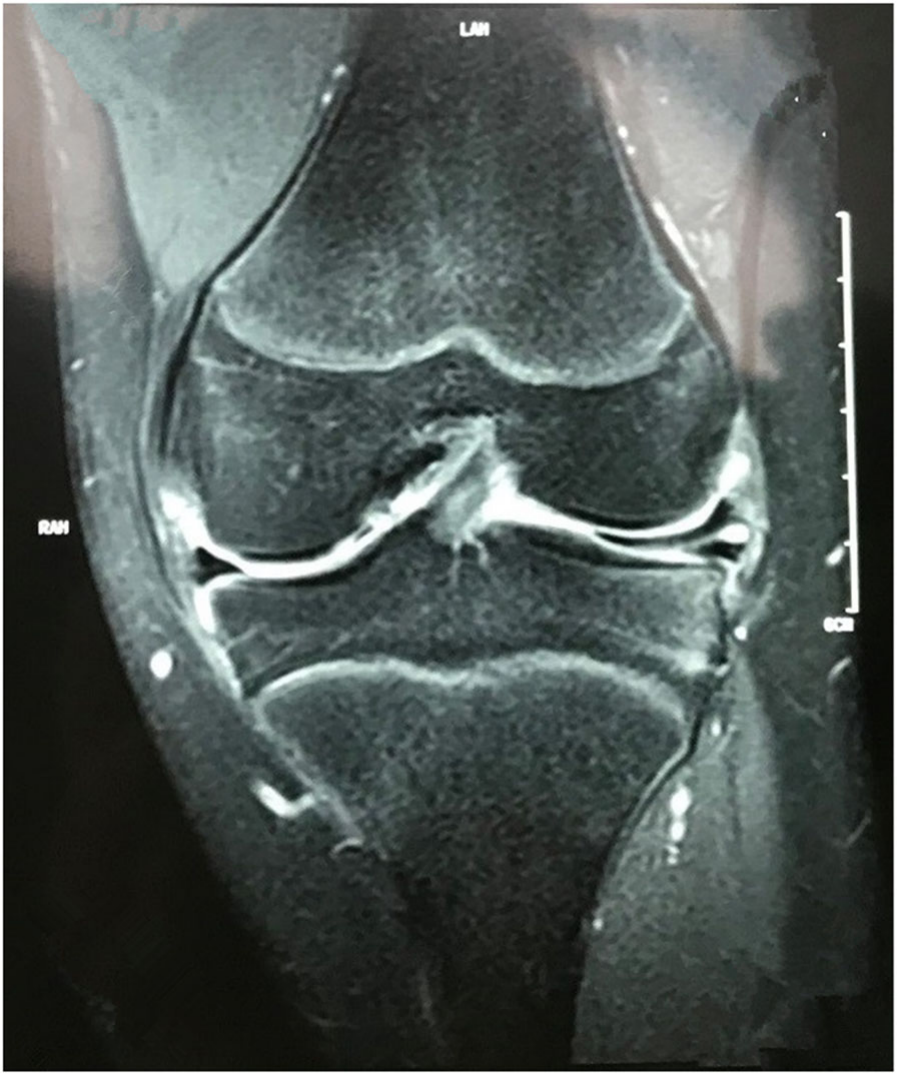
A horizontal tear of the lateral menisci, which is identified with high signal intensity in T2-weighted MRI
